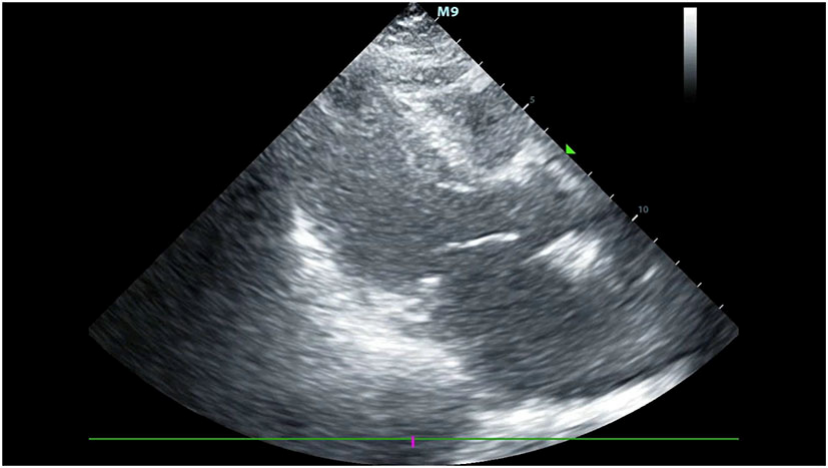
The bedside cardiac ultrasound suggested poor cardiac contractile function and left ventricular ejection fraction only 25%.
